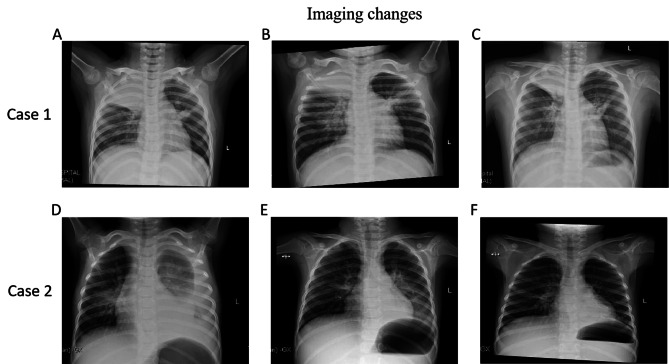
Imaging changes of case 1 and case 2. (A) Case 1: Chest radiography on day 3. (B) Chest radiography on day 6. (C) Chest radiography on day 10. (D) Case 2: Chest radiography on day 1. (E) Chest radiography on day 9. (F) Chest radiography on day 27
